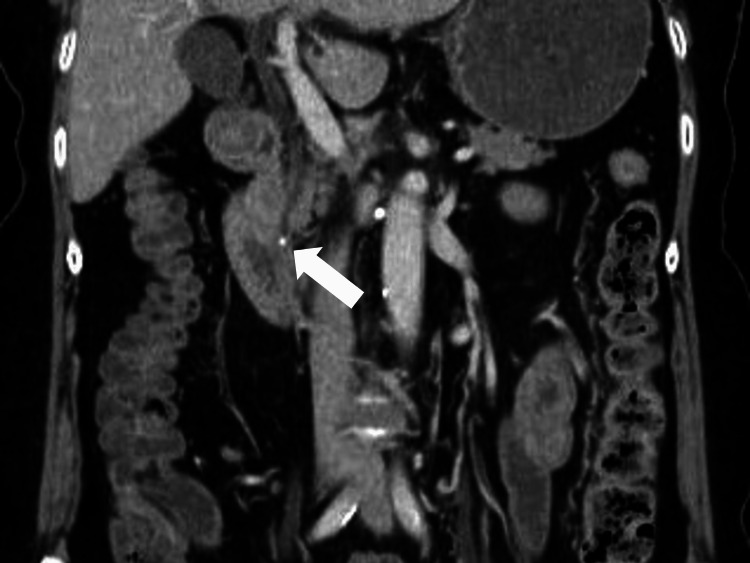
Abdominal computed tomography showing a stone in the lower bile duct (white arrow)
